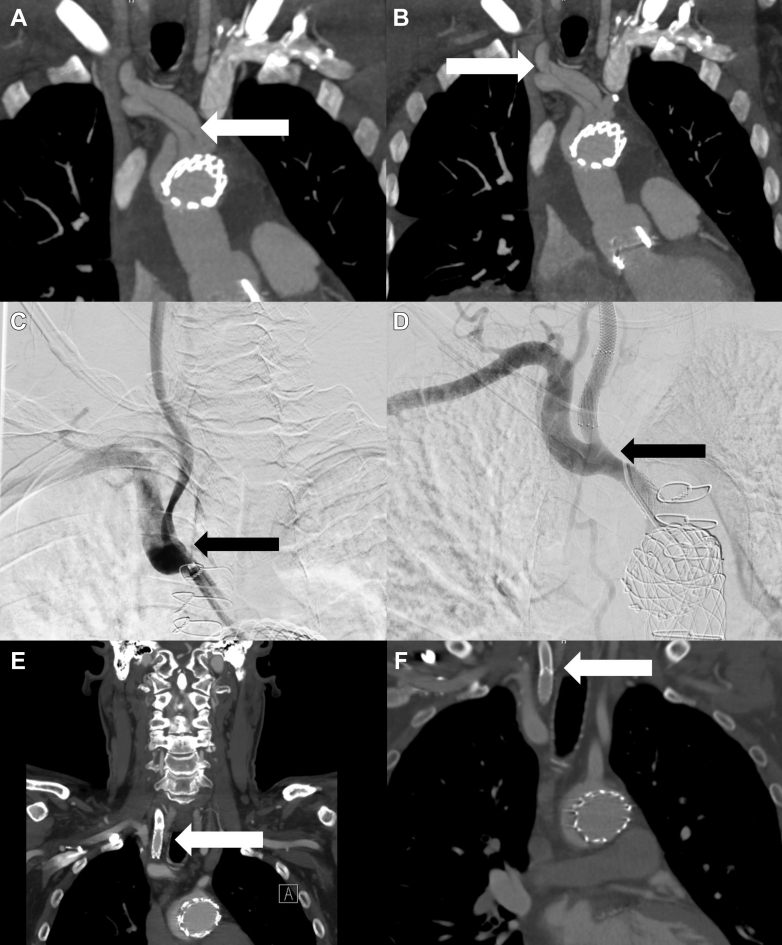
Postoperative Computed Tomography Scans Postoperative computed tomography (CT) demonstrating dissection of the innominate artery (A) and right common carotid artery (B), with re-entry tears present. Intraprocedural imaging from the head vessel stenting procedure demonstrating filling of the innominate artery false lumen prior to stenting (C), with resolution of false lumen filling poststenting (D). Postprocedure CT demonstrating a stent in the innominate artery (E) and right common carotid artery (F). Arrows indicate the area of interest.
